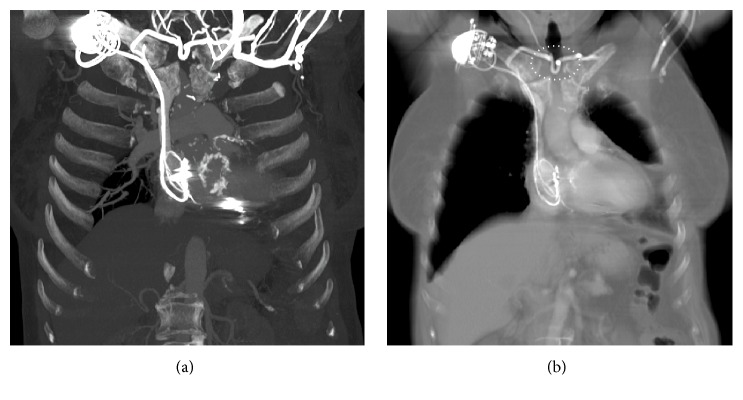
CT with administration of contrast media through a left peripheral vein. (a) Coronal maximum intensity projection image shows chronic occlusion of the left-sided central veins with cervical collateral veins from the left to the right side and here reaching the nonoccluded right-sided central veins; slight metal artifacts by the right-sided pacemaker. (b) Coronal multiplanar reconstruction image showing a partially quite tortuous u-shape horizontal-transverse midline vein in the jugulum (dotted ellipse), the AJVS with the JVA.
